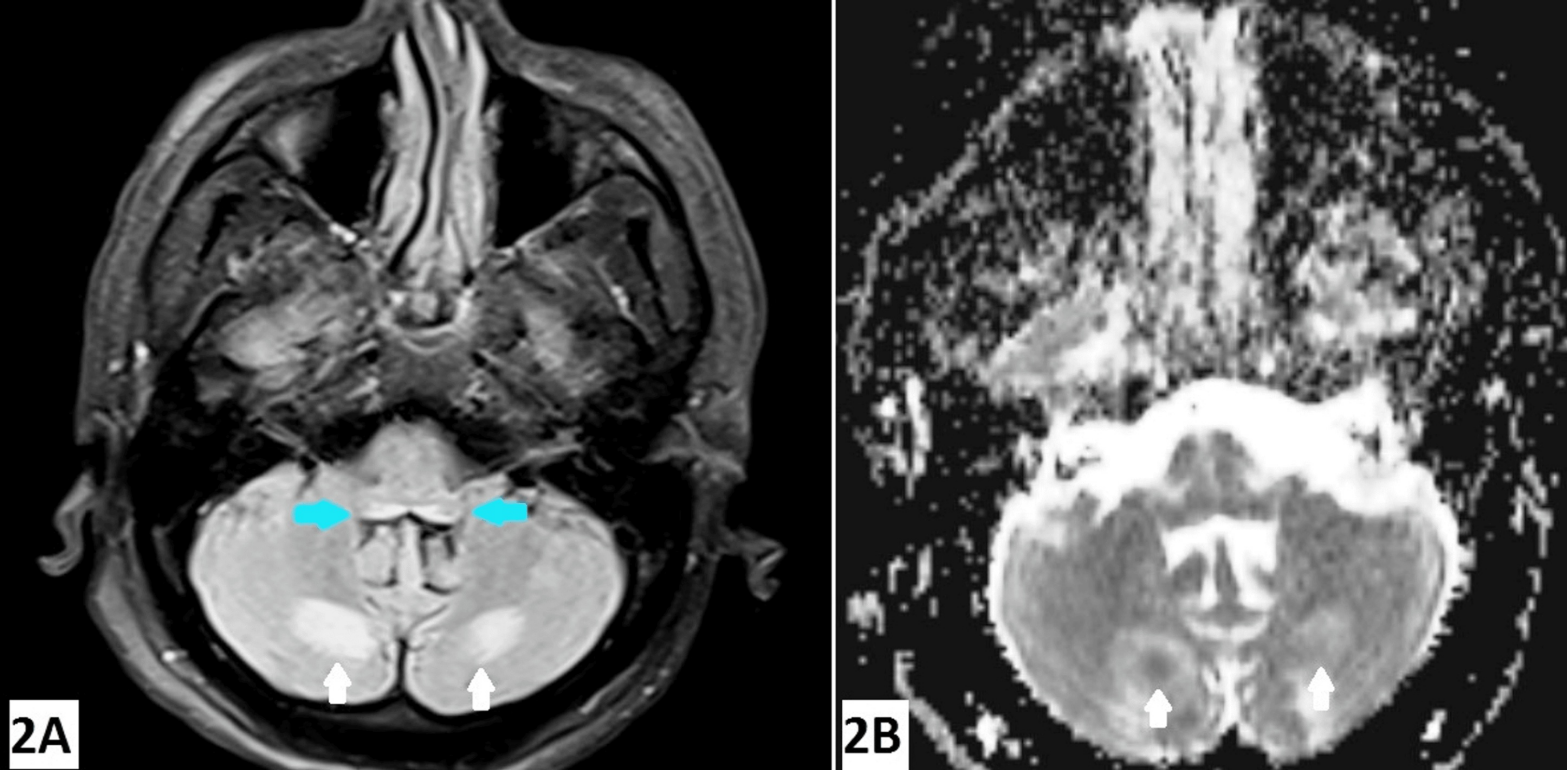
(A) Brain MRI (T2 FLAIR) showing areas of symmetrical FLAIR hyperintensities in an area posterior to the cerebellar dentate nuclei (white arrows) and dorsal aspect of the upper medulla (blue arrows) (magnification: 600 DPI). (B) Brain MRI ADC sequence showing areas of low ADC bilaterally in an area posterior to the cerebellar dentate nuclei (white arrows) (magnification: 600 DPI) FLAIR, fluid-attenuated inversion recovery; ADC, apparent diffusion coefficient
